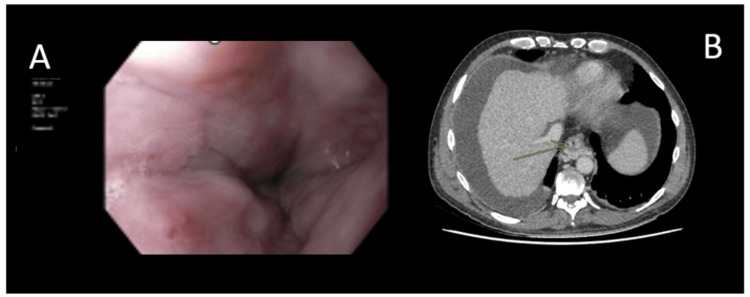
OGD (A) and corresponding portal phase contrast enhanced CT (B) of a patient with a red whale sign OGD: oesophagogastroduodenoscopy; CT: computed tomography
